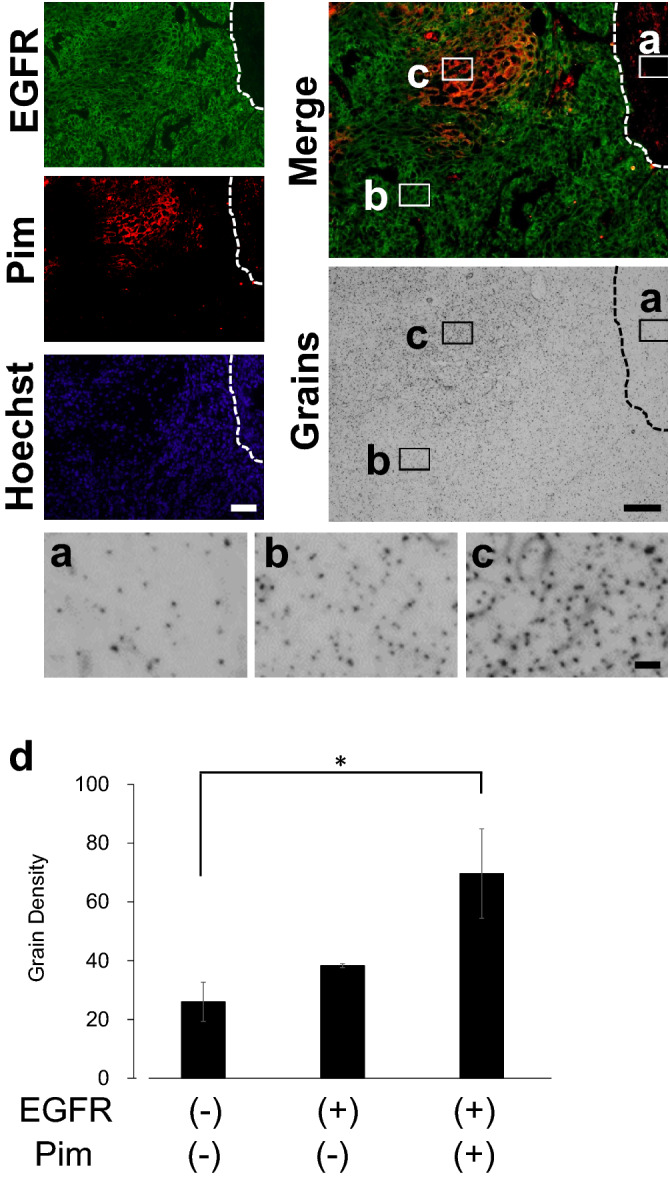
^18^F-FDG accumulation in hypoxic cells expressing EGFR. The dotted line shows the boundary between the tumor and the skin area. (a) Magnified view of the micro-autoradiography image showing the pimonidazole- and EGFR-negative skin area, (b) pimonidazole-negative and EGFR-positive area, and (c) pimonidazole- and EGFR-positive area. (d) Determination of grain density in the pimonidazole- and EGFR-positive and negative areas, focusing on the same-sized ROIs (n = 3, mean ± SEM, Tukey’s multiple comparison, *: *p* < 0.05). Pim: Pimonidazole. Bars: 100 μm (EGFR, Pimonidazole, Hoechst, Merge and Silver grains), 10 μm (a–c).
